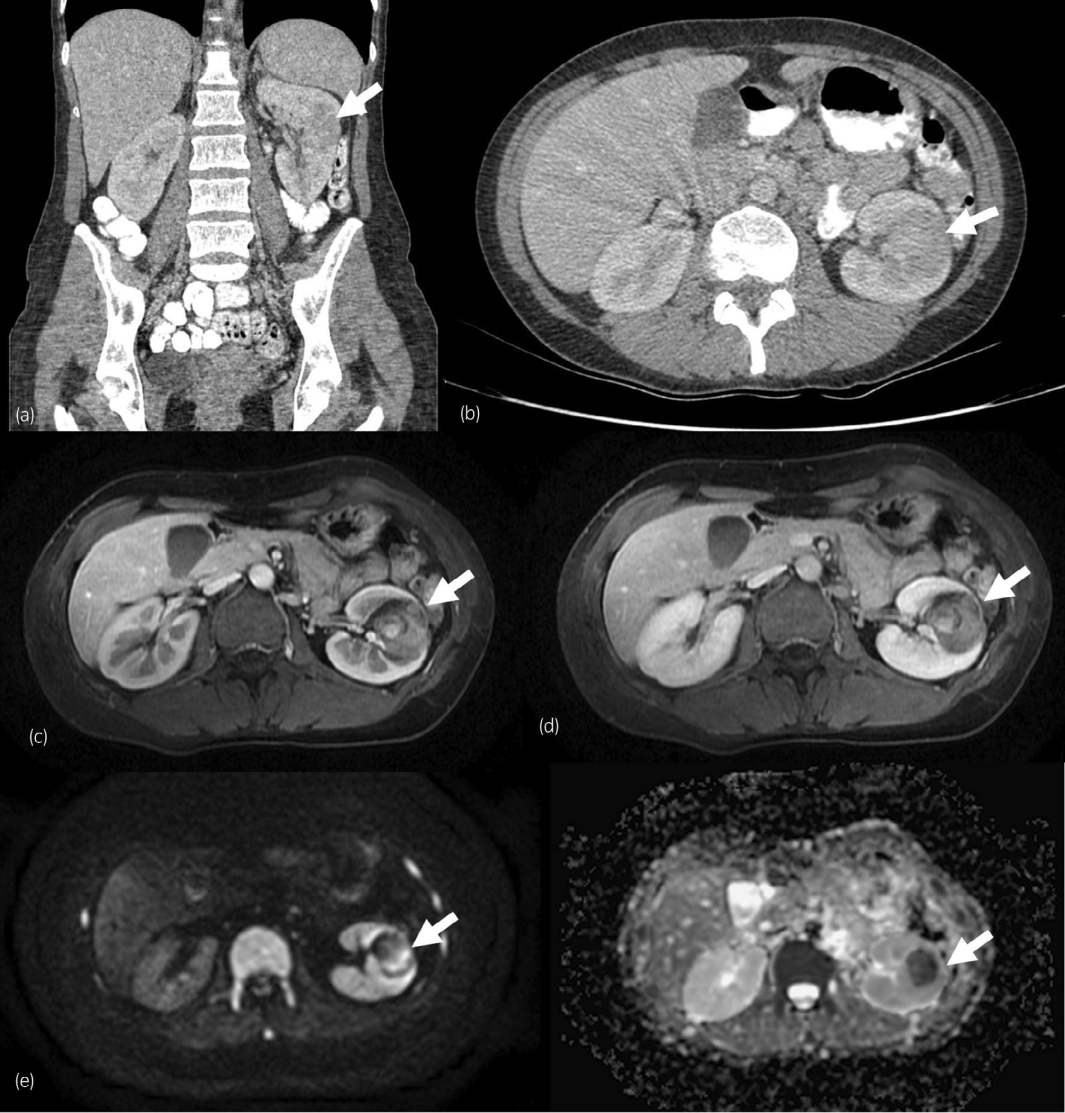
Solid mass on the left kidney. (a) CT portal venous phase, coronal image; (b) CT portal venous phase, axial image; (c) MRI arterial phase, axial image; (d) MRI venous phase, axial image; (e) MRI DWI/ADC showing diffusion restriction of the lesion, axial image.
